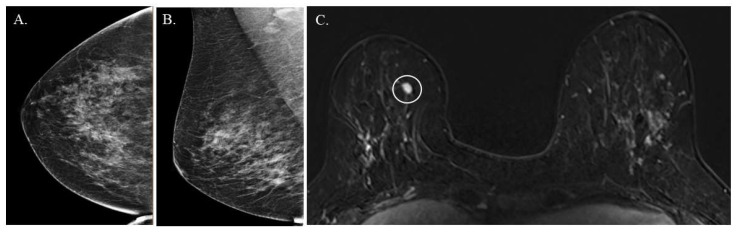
A 50-year-old female with calculated TCv8 lifetime risk of 26.5% undergoing annual mammography and supplemental screening breast MRI in a concurrent regimen. (A,B) Right breast screening mammogram with craniocaudal (CC) and mediolateral oblique (MLO) views demonstrates category C tissue density (heterogeneously dense) without suspicious abnormality. (C) Breast MRI, axial post-contrast, subtraction image of the inner right breast shows a 7 mm irregular enhancing mass and mild background parenchymal enhancement (circle). Biopsy confirmed invasive ductal carcinoma.
